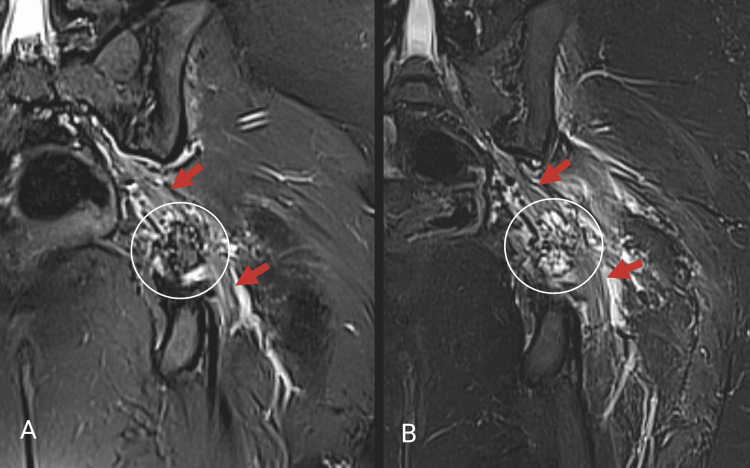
Coronal T2-weighted images of the left sciatic nerve lesion at six-month follow-up. (A) Initial coronal T2 image demonstrates the lesion (white circle), with a hypointense rim consistent with hemosiderin deposition. The sciatic nerve shows thickening along its course (red arrows). (B) Follow-up T2 image at six months shows lesion progression (white circle) and thickening along the sciatic nerve (red arrows).
